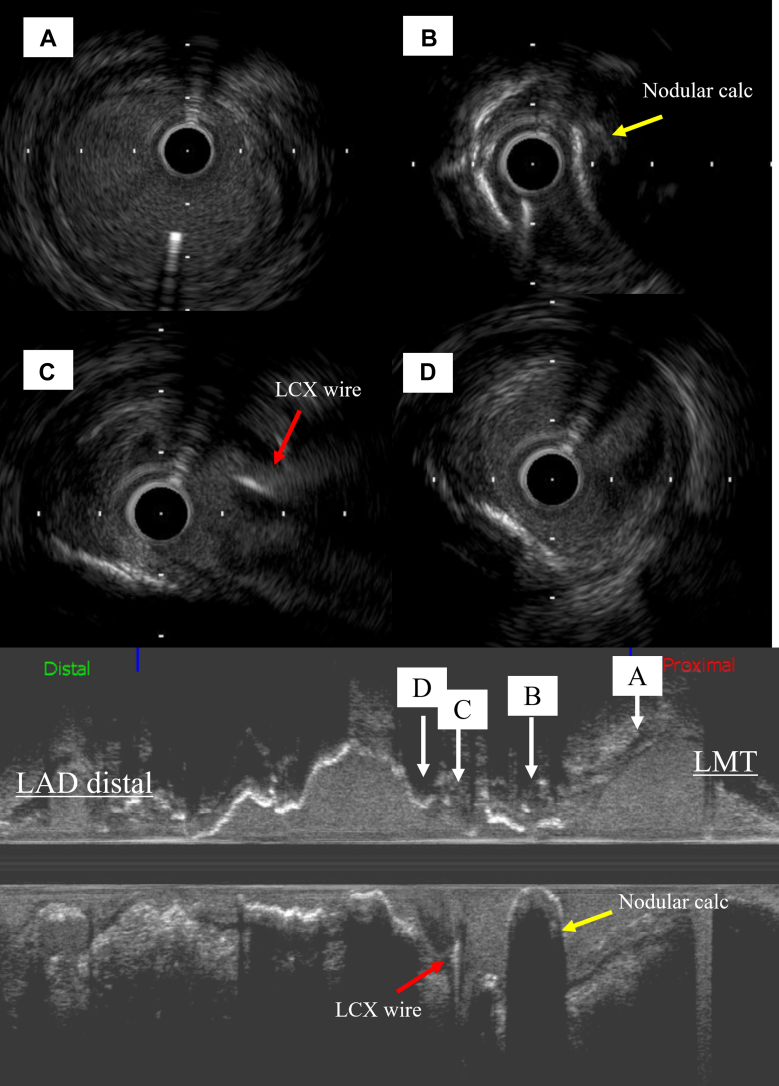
Intravascular Ultrasound Images (A) Ostial left coronary artery. (B) An eccentric calcified lesion with acoustic shadowing. (C) Bifurcation of the distal LMT to the circumflex artery. Mild atherosclerotic plaque is noted from the 9 o'clock to 1 o'clock direction. (D) Just proximal of the LAD. calc = calcification; LAD = left anterior descending artery; LCX = left circumflex artery; LMT = left main trunk.
